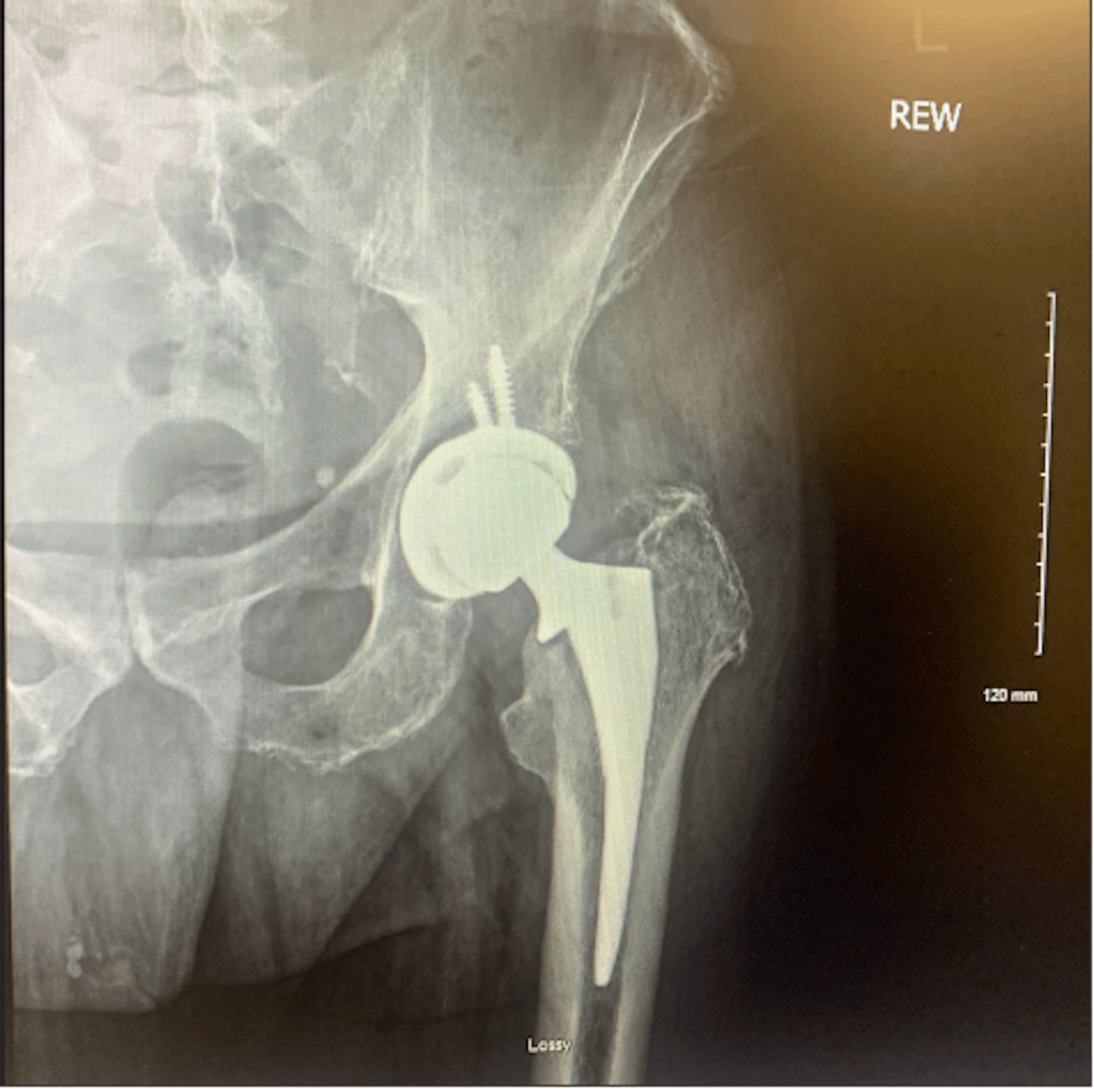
Postoperative anteroposterior radiograph of the left hip
